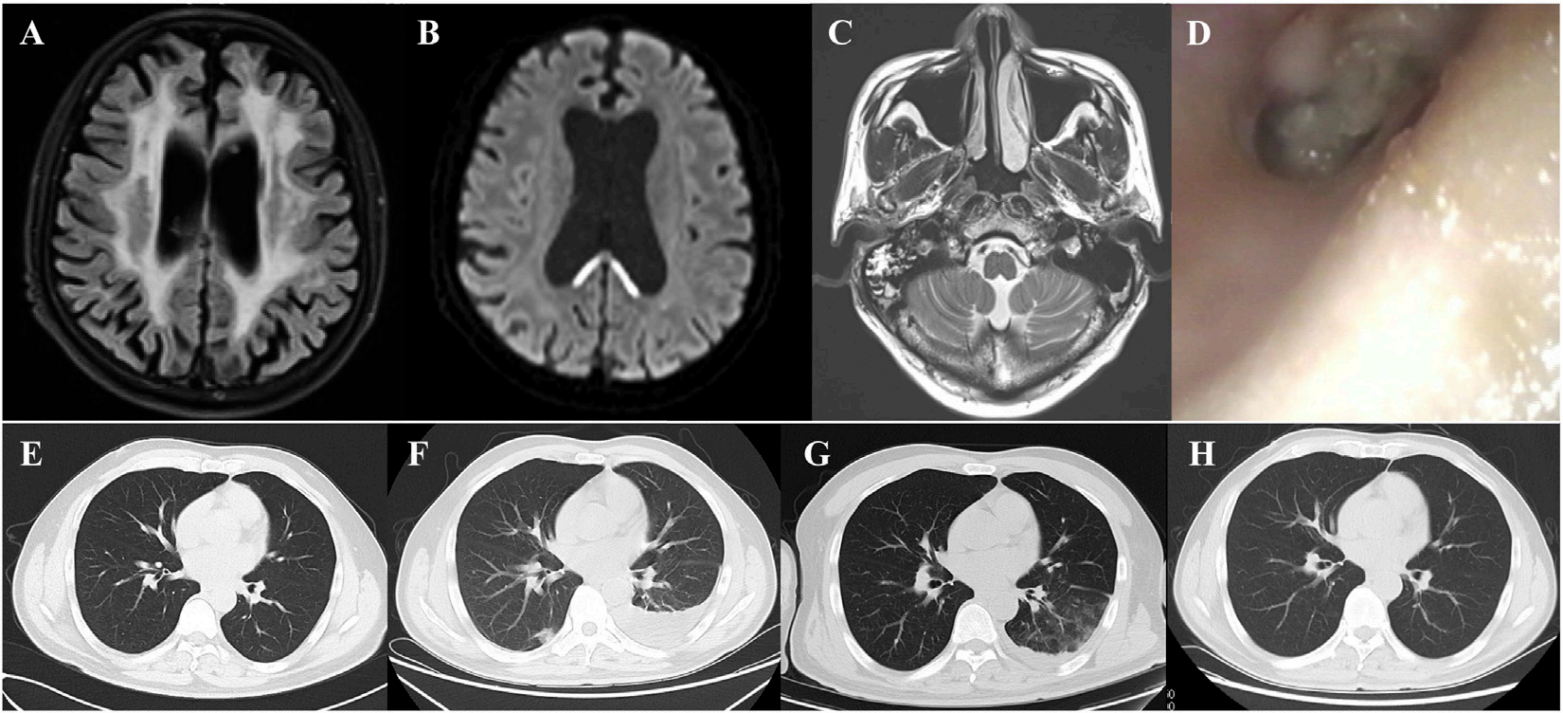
Radiographic and clinical findings during disease progression and infection events. (A,B) Brain MRI at initial presentation: T2-FLAIR images showing bilateral periventricular white matter hyperintensity and thinning of the corpus callosum, suggestive of hereditary leukoencephalopathy. (C) MRI of the temporal bone on day +16: inflammation of the right mastoid air cells consistent with otomastoiditis. (D) Otoscopic image showing purulent discharge in the right external auditory canal. (E) Chest CT on admission: no pulmonary abnormalities. (F) Chest CT on day +49: bilateral patchy infiltrates indicating fungal pneumonia. (G) Thoracentesis on day +63: turbid brown pleural fluid suggestive of fungal empyema. (H) Chest CT on day +85: resolution of prior lesions, with residual pleural effusion.
